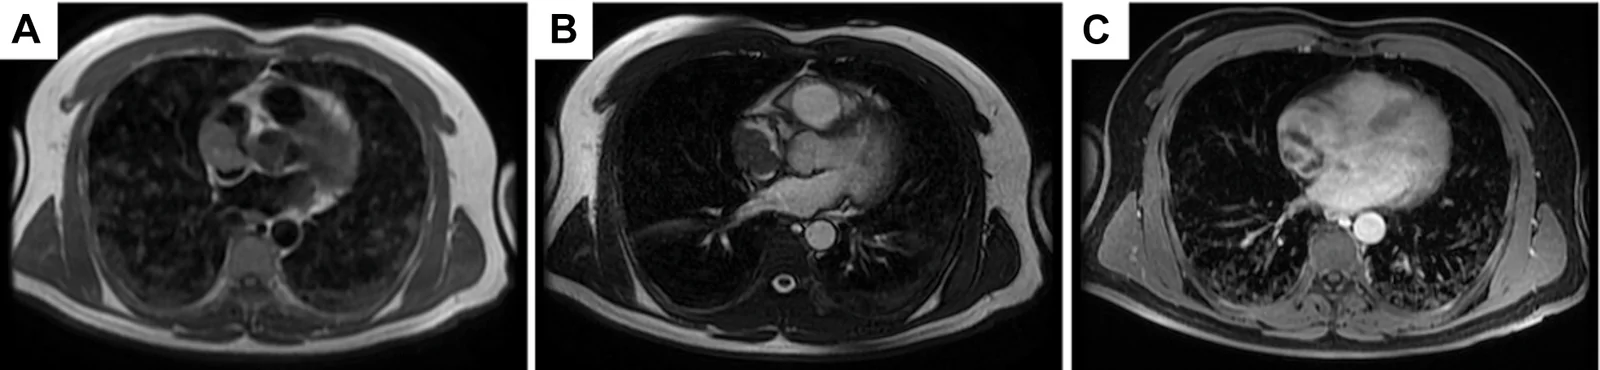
A-C, Cardiac MRI scan in 8-mm slices with black blood images (A) and fast imaging using steady-state acquisition short-axis images (B) demonstrated minimal heterogeneity in signal centrally. C, Postgadolinium enhancement was also heterogeneous centrally, consistent with a solid enhancing mass with likely some associated peripheral hemorrhage.
